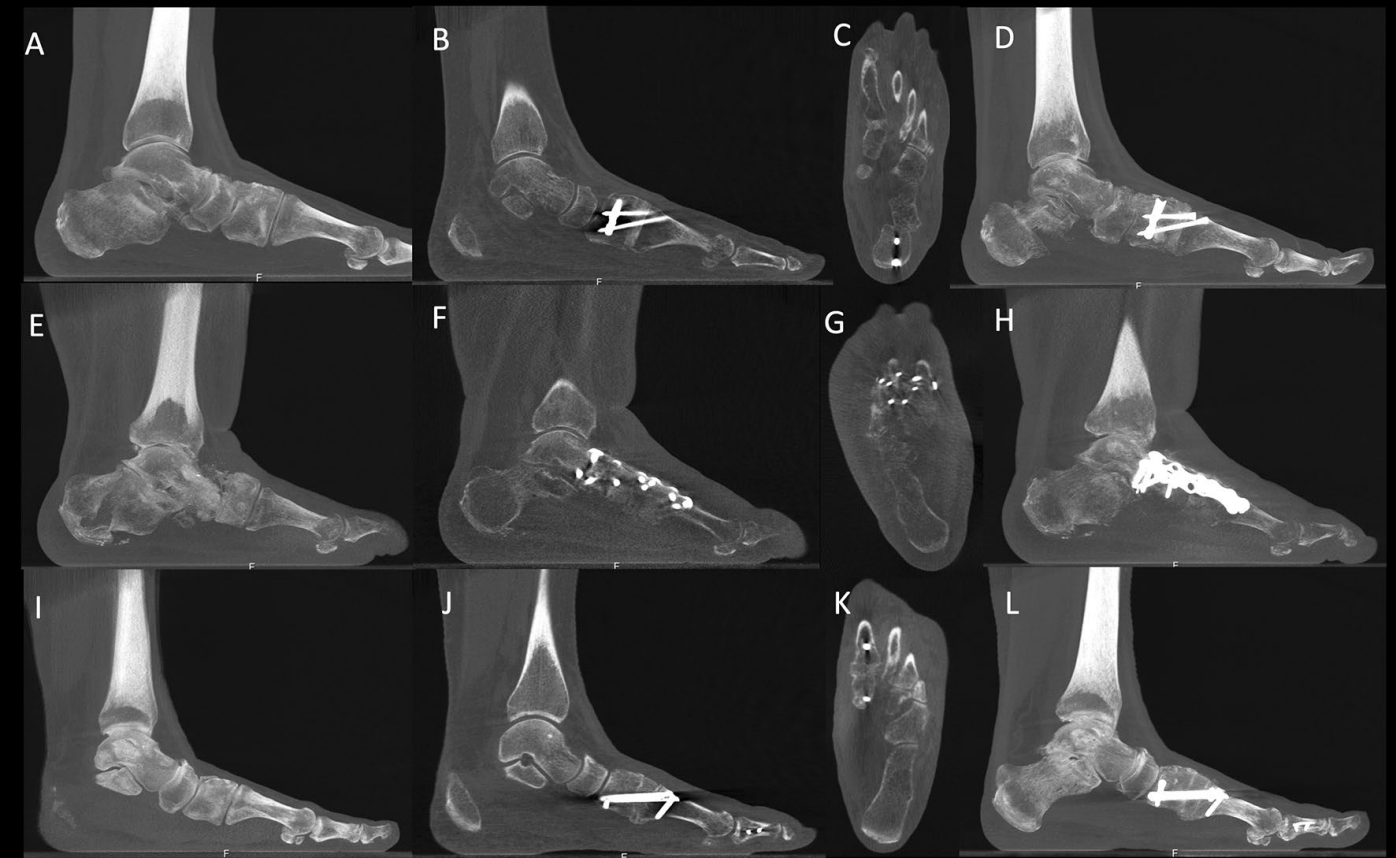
Examples of preoperative and postoperative weight-bearing CT (WBCT) images for the three different types of implants utilized for fixation of the LapiCotton procedure (A–D medial cuneiform post; E–H plate and screws; I–L intramedullary device). First column on the left (A, E and I) shows a thick sagittal cut of the preoperative deformity. Second (B, F and J) and third columns (C, G, and K) demonstrate, respectively, sagittal and axial 3-month postoperative WBCT imaging of the first tarsometatarsal fusion site, with full integration of the allograft and complete bone healing with all three different implants. Last column on the right (D, H and L) shows a thick sagittal WBCT image of the postoperative construct
